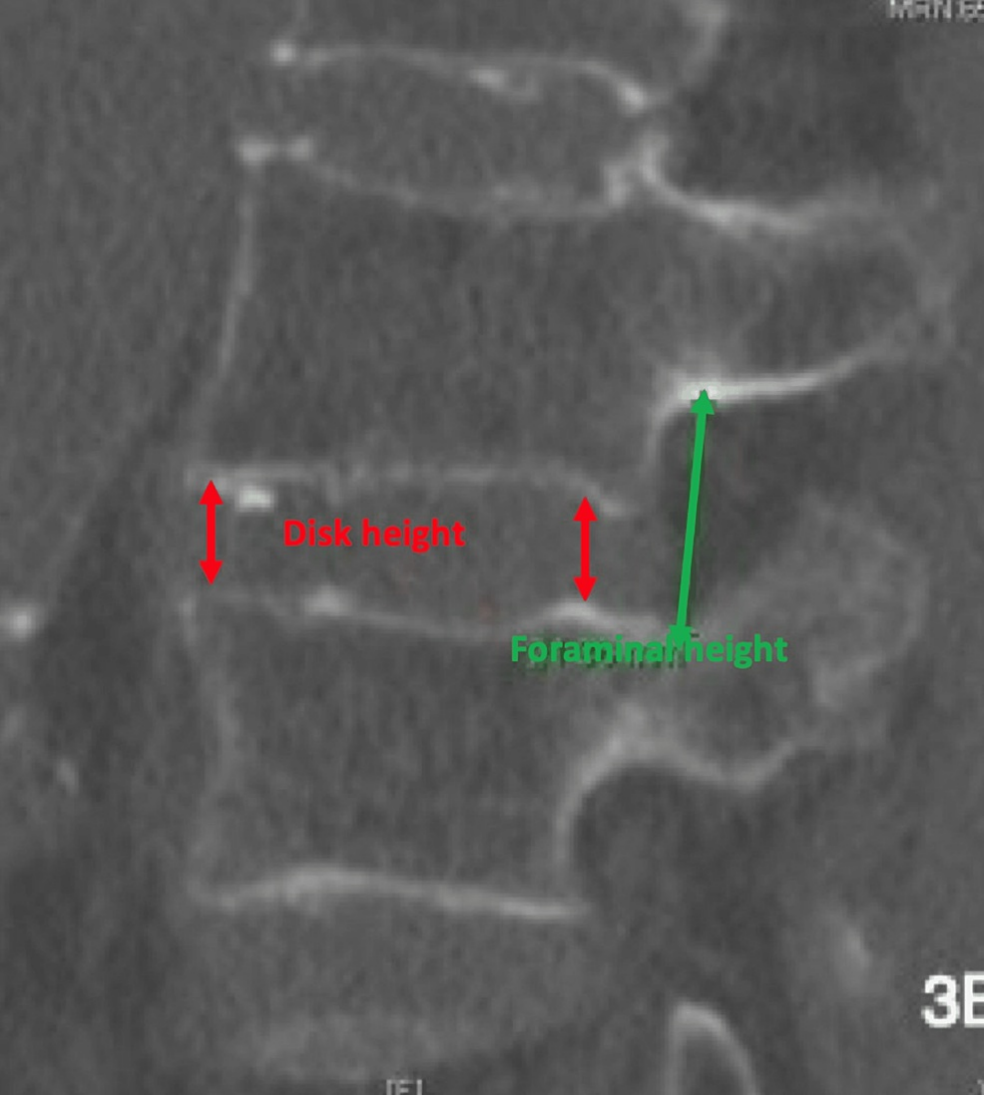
The disc height was measured as the distance between the caudal vertebra's upper surface and the lower surface of the cranial vertebra at the anterior (anterior disc height) and the posterior border (posterior disc height). The average between both was calculated (average disc height). The foraminal size was measured as the distance between the caudal pedicle's upper edge and the lower edge of the cranial pedicle. Measurement of the lumbar CT scan sagittal view is demonstrated.
